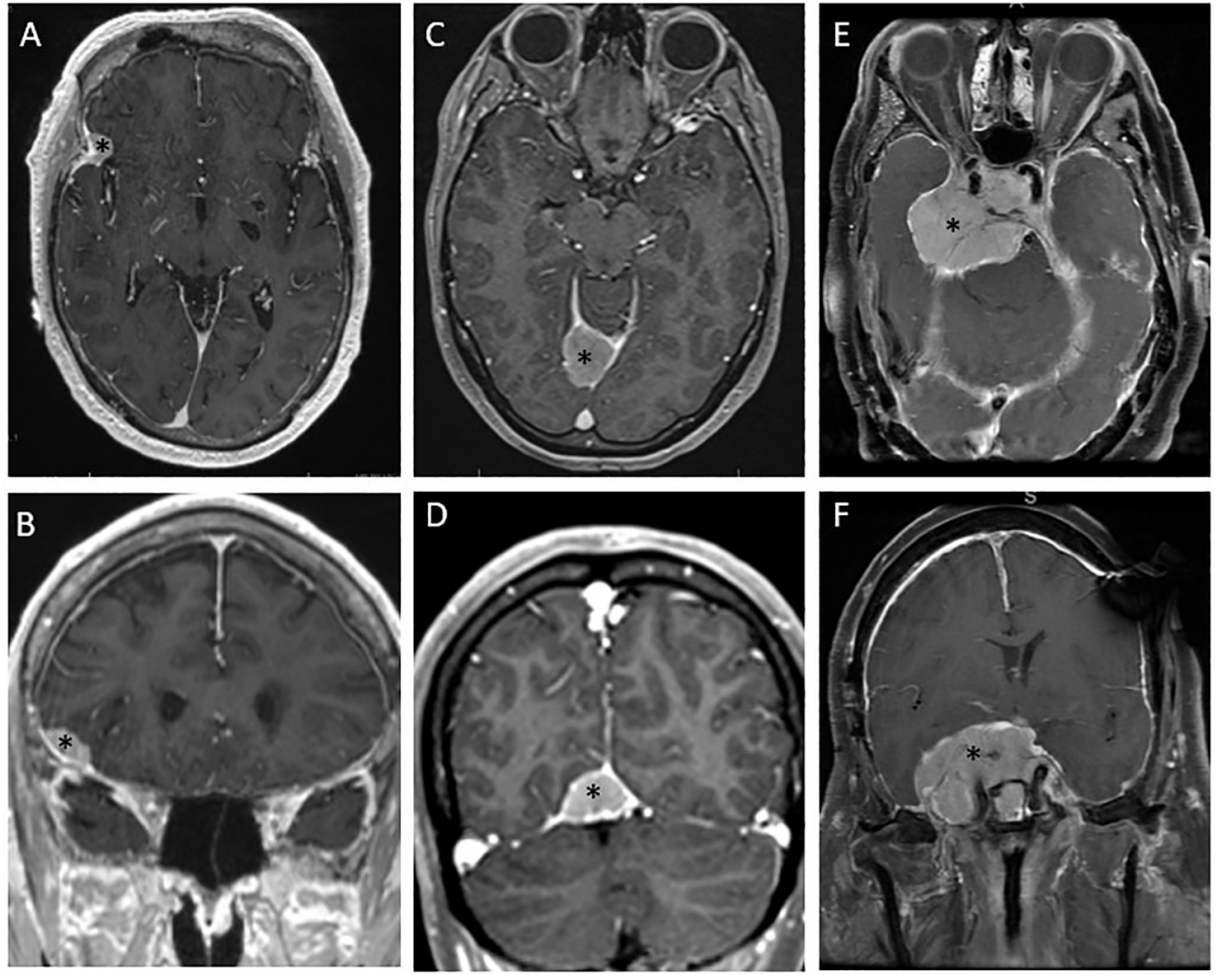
Diverse presentations of and management strategies for meningiomas. (A, B) Axial and coronal magnetic resonance (MR) images depict a presumed grade 1 meningioma incidentally discovered on a fluciclovine positron emission tomography (PET) scan for metastatic prostate cancer. The low-risk location was thought to make this lesion amenable to observation, and the lack of growth on the 2-month MR images increased confidence in the diagnosis of low-grade meningioma as opposed to dural-based metastasis. This lesion was considered appropriate for observation. (C, D) Axial and coronal MR images depict a tentorial meningioma that had previously been subtotally resected. This lesion continued growing after resection and was then treated with single-fraction radiosurgery. (E, F) Axial and coronal MR images depict a large suprasellar base-of-skull meningioma involving the right cavernous sinus and encasing the optic chiasm with a mass effect on the brainstem. This lesion had been subtotally resected 5 years previously and grew very slowly, eventually causing visual field cut. This lesion was treated with conventionally fractionated radiotherapy. *denotes the location of the tumor.
